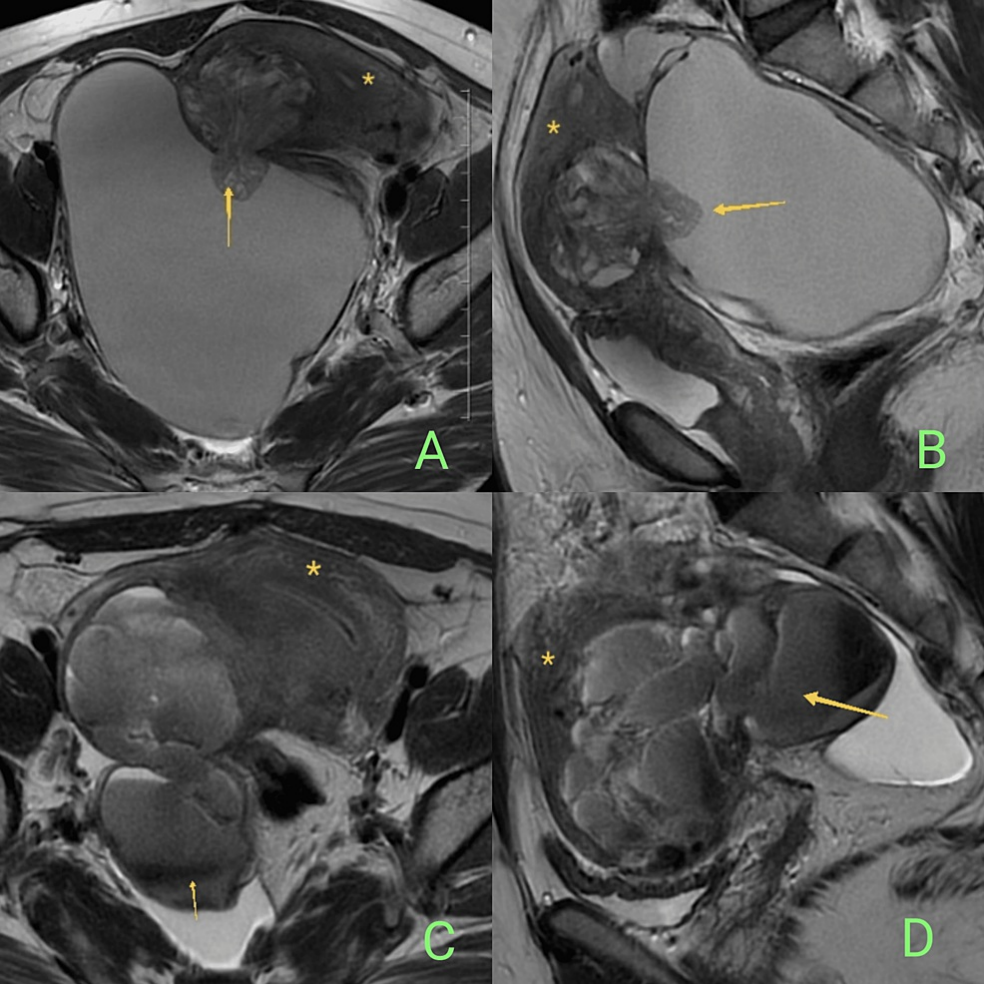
MRI findings of exophytic adenomyoma MRI T2-axial (A) and sagittal (B) images during the initial presentation depicting an exophytic adenomyomatous polyp (arrow) extending from the right posterolateral wall of the uterus (asterisk) abutting into the right endometriotic cyst. The JZ is intact. MRI T2-axial (C) and sagittal (D) images during the recurrence depict a cystic adenomyoma (arrow) at the same site with a posterior serosal breech communicating with the right endometriotic cyst.
